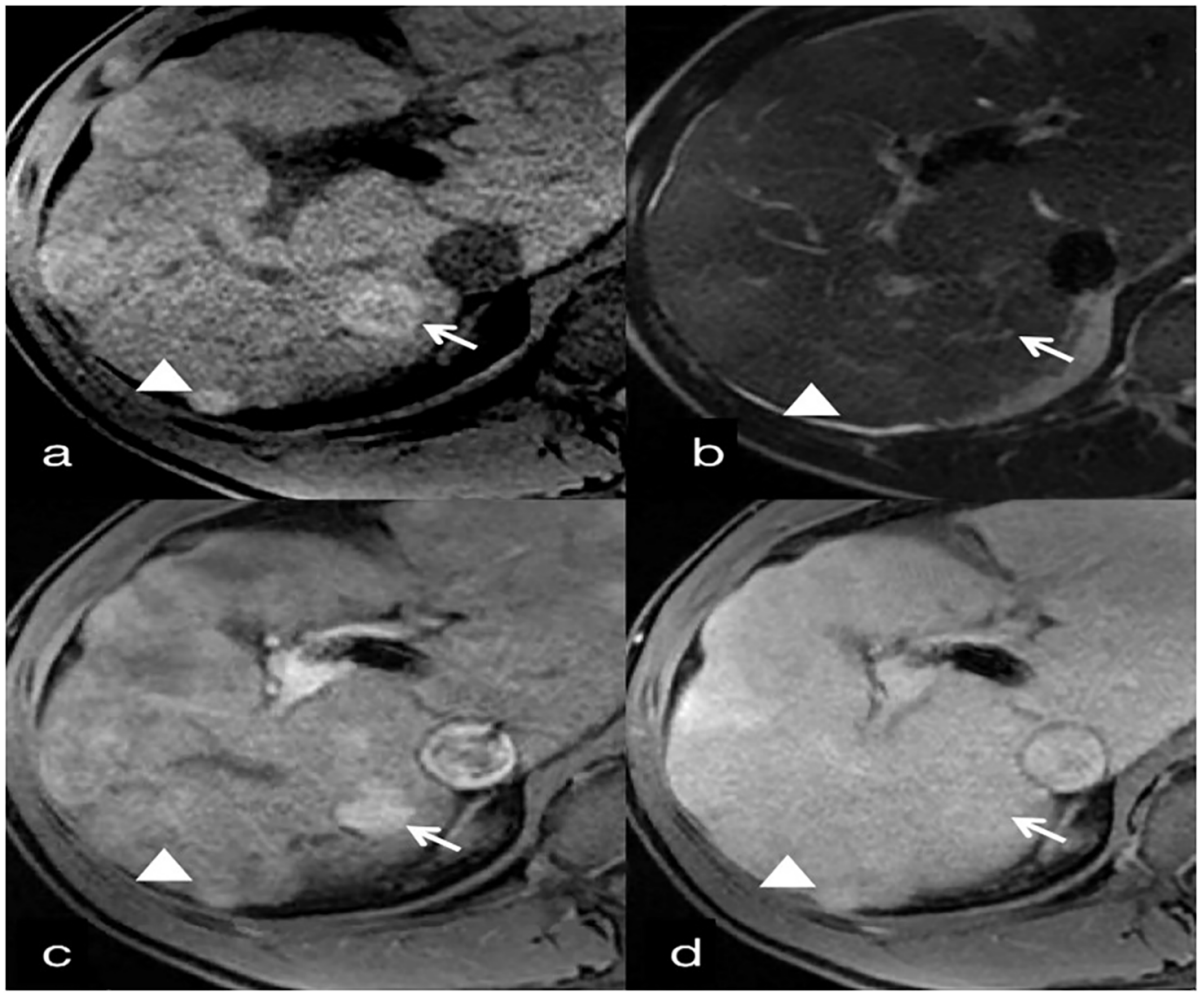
30-year-old man with benign hepatocellular lesions associated with Budd-Chiari syndrome. (A) T1-weighted image shows hyperintense benign hepatocellular lesions (arrow) with an internal hypointense scar. A second benign hepatocellular lesion (<2cm) under the liver capsule is homogeneously hyperintense (arrowhead). (B) T2-weighted image shows mildly hypointense benign hepatocellular lesions compared to surrounding liver parenchyma, with an internal small hyperintense scar (arrow). A second benign hepatocellular lesion (<2cm) is homogeneously mildly hypointense (arrowhead). (C) Contrast-enhanced T1-weighted image obtained 20 sec (arterial phase) after initiation of contrast agent administration shows homogeneous hyperenhancement of both lesions, which are slightly hyperintense in the venous phase (D).
